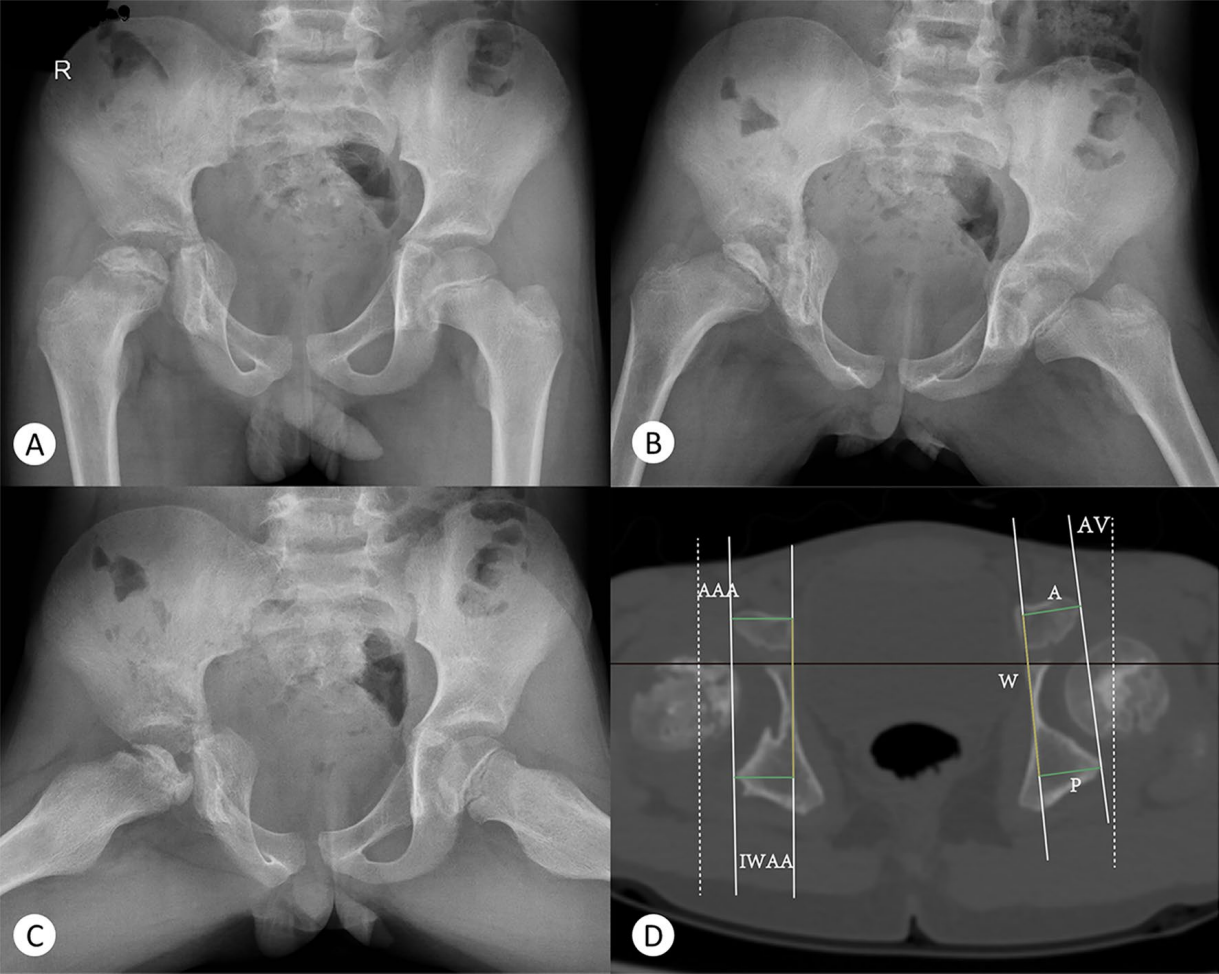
An eight-year-old boy with Legg-Calve-Perthes disease in the right hip. The anteroposterior pelvis radiograph (A), Von-Rosen pelvis radiograph (B) and frog pelvis radiograph (C) show that patient was in Waldenstrom II stage, Herring B stage and Catterall III stage.
